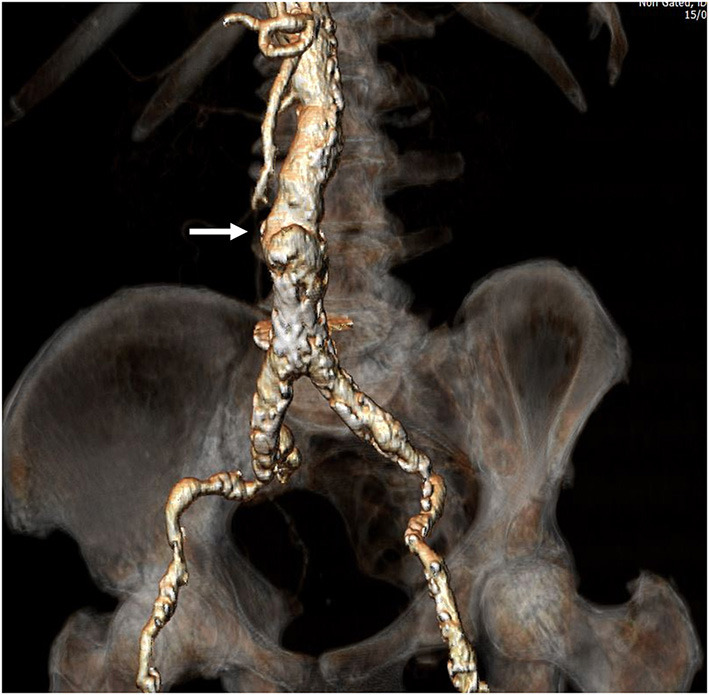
Preprocedural computed tomography angiography. Full evaluation of bilateral ilio-femoral arteries was performed assessing for minimal vessel diameter, extent and circumference of calcification, and vessel tortuosity. Once a patient was deemed as high-risk for standard trans-femoral access as shown in the figure, a calcium-free window in the infra-renal abdominal aorta was identified. This would be the target for traversal from the vena cava to the abdominal aorta (Arrow).
